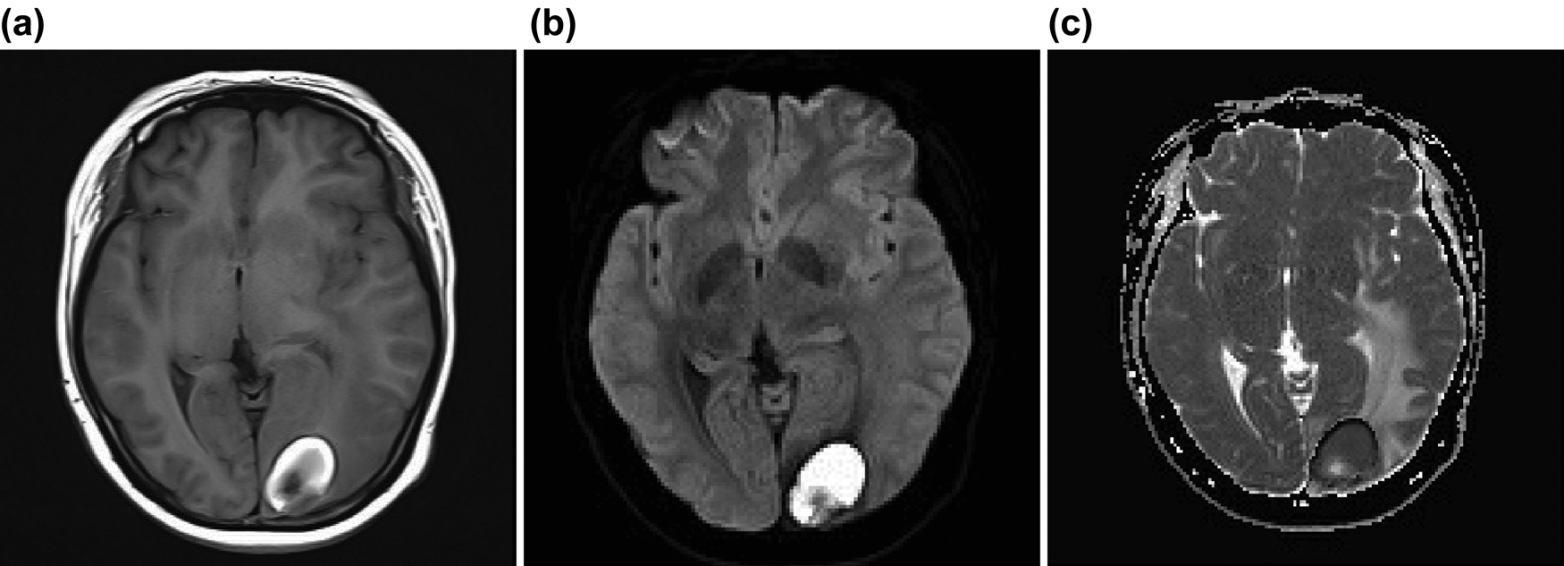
DCE-MRI, DWI, and ADC before treatment. Note: The depicted patient was male, 61 years old, with no smoking history, diagnosed with brain metastasis of squamous adenocarcinoma. (a) DCE-MRI image, showing a ring enhancement lesion about 4.3 cm in the right temporal lobe, with significantly low signal in the center (where the arrow points); (b) DWI image, showing significantly low signal in the center of the lesion, equal or high mixed signal at the edge, and equal signal in peritumor edema; (c) ADC image, showing accelerated diffusion of the lesion center and unrestricted diffusion of surrounding edema.
